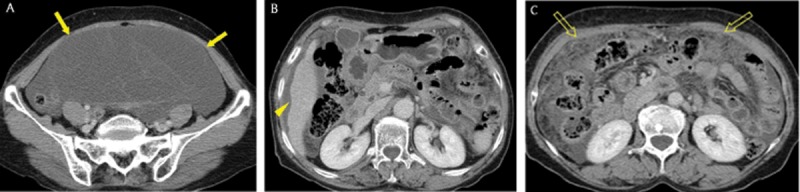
A 66-year-old woman with pseudomyxoma peritonei caused by appendiceal mucinous carcinoma. (A, B) Axial portal venous phase CT images showed fluid collection (arrows) with septa in the peritoneal cavity. Note scalloping of the liver surface (arrowhead) which implied extrinsic pressure by fluid in the perihepatic space. (C) Omental haziness (open arrows) was also detected.
